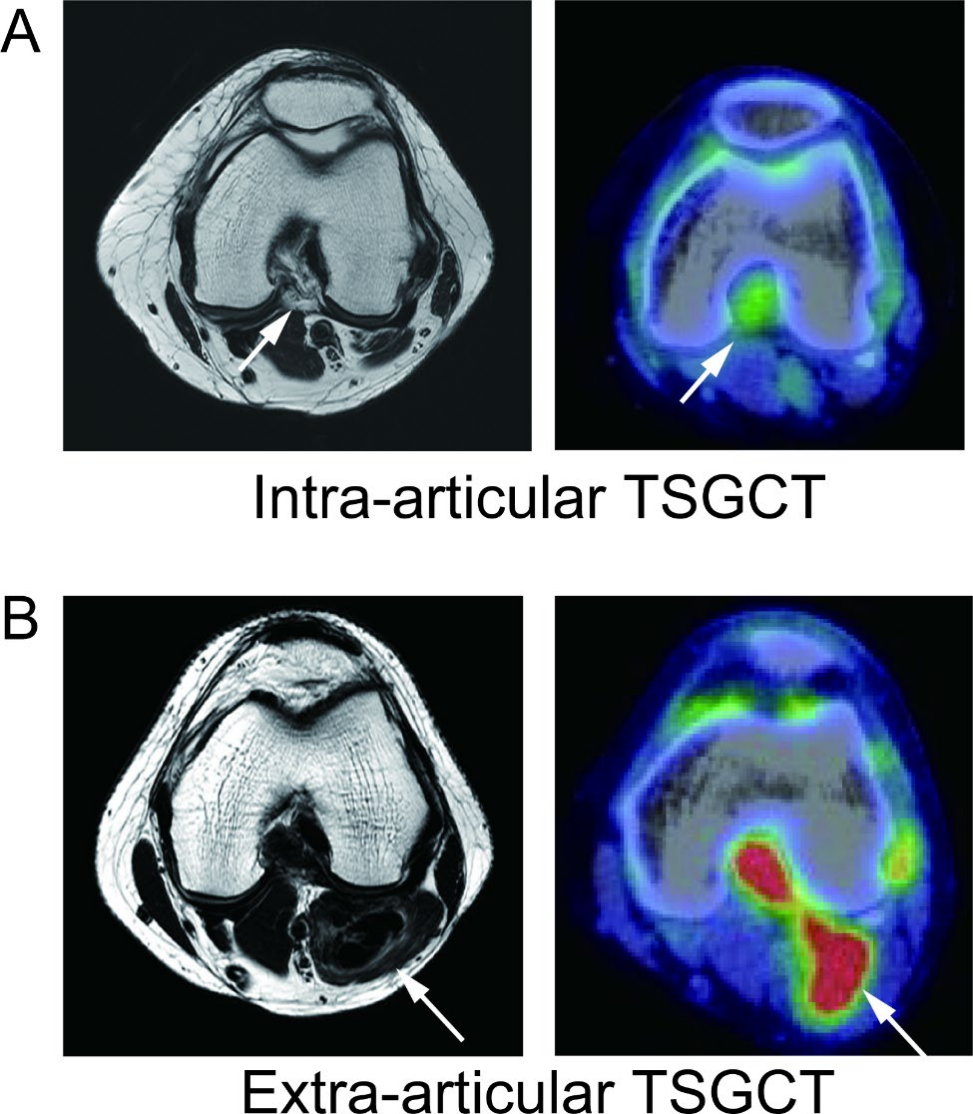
Representative magnetic resonance imaging (MRI) and positron emission tomography/computed tomography (PET/CT) fused images of intra- and extra-articular tenosynovial giant cell tumor. (A) Intra-articular tenosynovial giant cell tumor (*arrow*). *left*, T2 weighted axial view of MRI; *right*, PET/CT fused image (Case No. 5 in dataset). (B) extra-articular tenosynovial giant cell tumor (*arrow*). *left*, T2 weighted axial view of MRI; *right*, PET/CT fused image (Case No. 12 in dataset)
